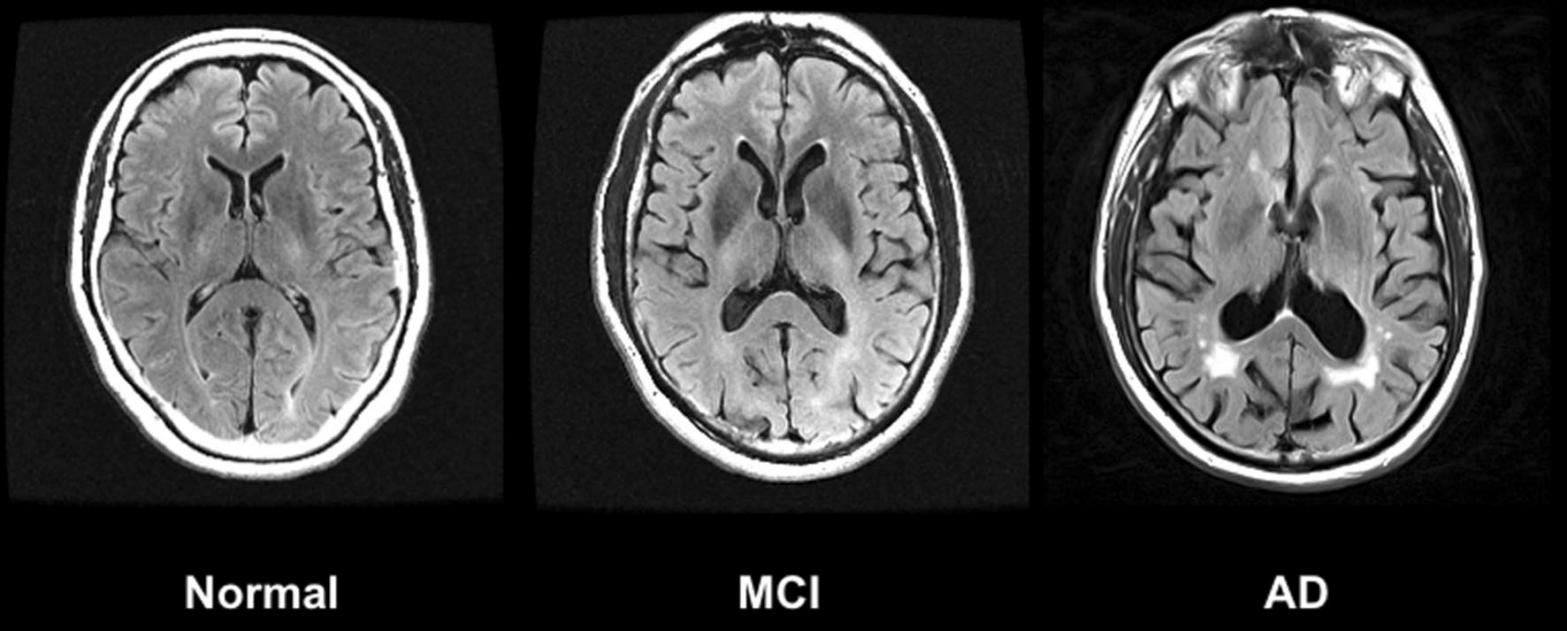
T2-weighted MRI imaging using a FLAIR (Fluid Attenuated Inversion Recovery) sequence shows increased WMHs in an AD patient compared to a healthy control and intermediate levels of WMHs in a patient with MCI
